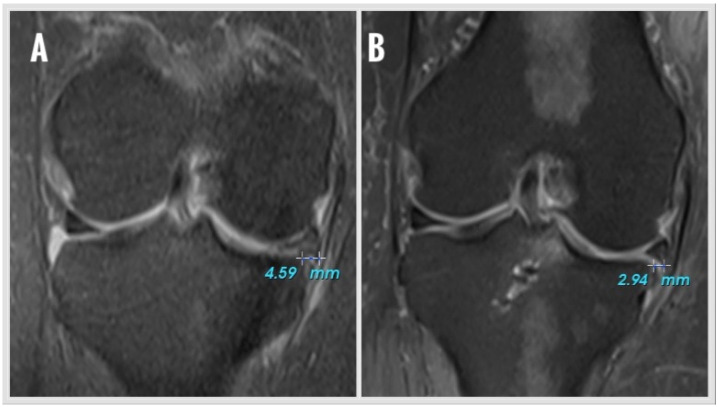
Meniscus extrusion (ME) measurement on coronal MRI section showing the most prominent appearance of the tibial eminence. (A) preoperative ME value, (B) postoperative ME value.
